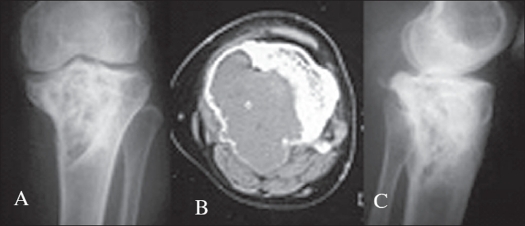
Campanacci Stage 3. This case was treated by curettage and bone grafting in 1997. A) CT scan before surgery. B) and C) X-ray taken in 2003. Review in 2005 shows no evidence of recurrence
